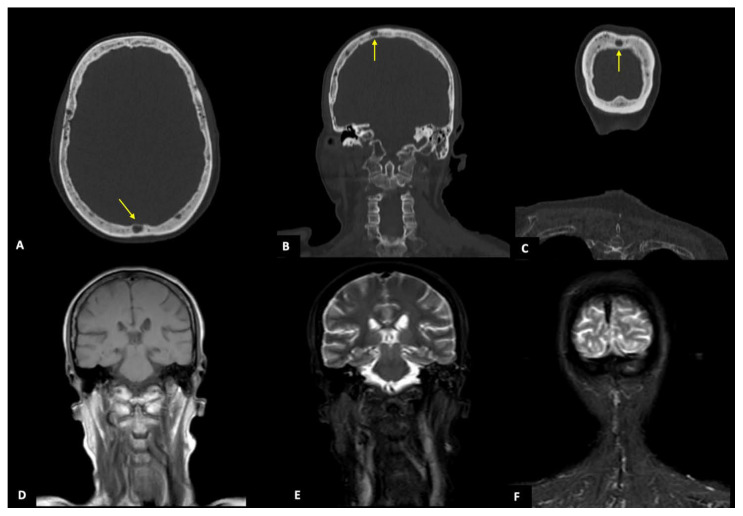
CT and MRI images from a 64-year-old woman with a focal infiltration pattern. (A–C): WBLDCT axial section and MPR images in coronal view showing some focal lytic lesions of the skull of whom the biggest (15 mm) is highlighted by the yellow arrow. The bone lytic lesions are not recognizable at all in image (D), a coronal T1-weighted image of the skull nor in images (E,F), and coronal STIR-weighted images of the skull.
